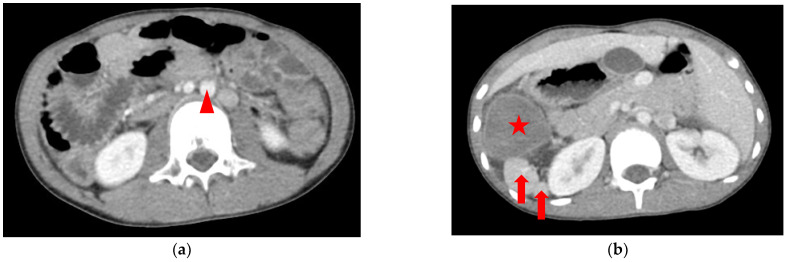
CT of the abdomen showing that the liver is full in the midline of the abdomen: (a) the hypodense tumor is located in the right abdomen <star>, and the superior mesentery vein <arrow head> lies to the left of the superior mesentery artery; (b) seven arrowhead spleens <arrows> are suspected beside the tumor (all spleens cannot be shown in two pictures). See the complete series of CT in Supplementary Materials Figure S1: CT of abdomen.
